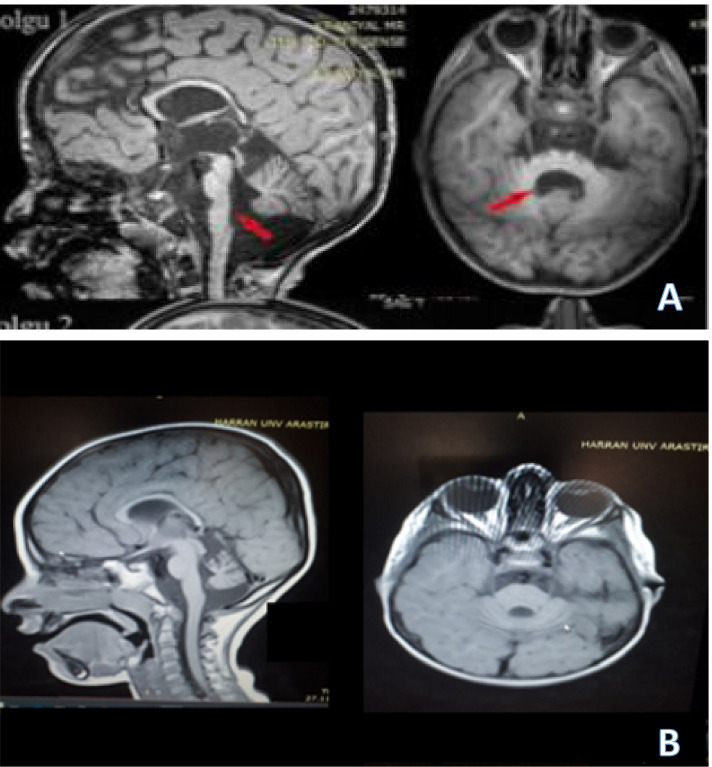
A 8 years old boy with PCH type 11, brain MRI ( sagittal and axial sequences) is showing, MRI-Ventral pons flattening, vermis hypoplasia ( blue arrow). Minimal hypoplasis of the hemispheres. B 7 years old boy with PCH type 7 brain MRI( sagittal and axial sequences) is showing Vermis hypoplasia. hypoplastic hemispheres, corpıs Callosum hypoplasia, and cortical atrophy
